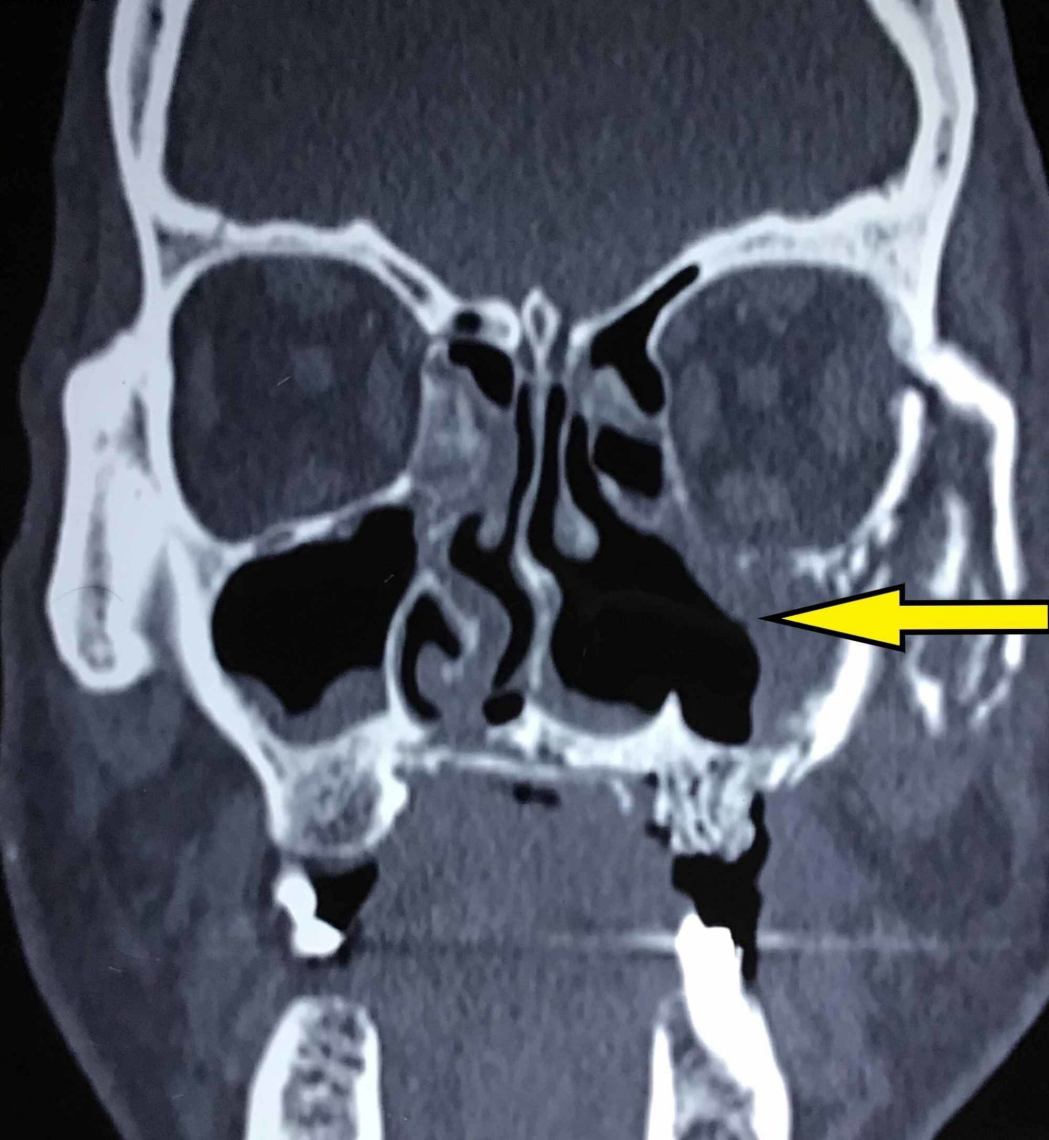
Postoperative CT at three months follow up revealing resolution of sinus obliteration following treatment.
